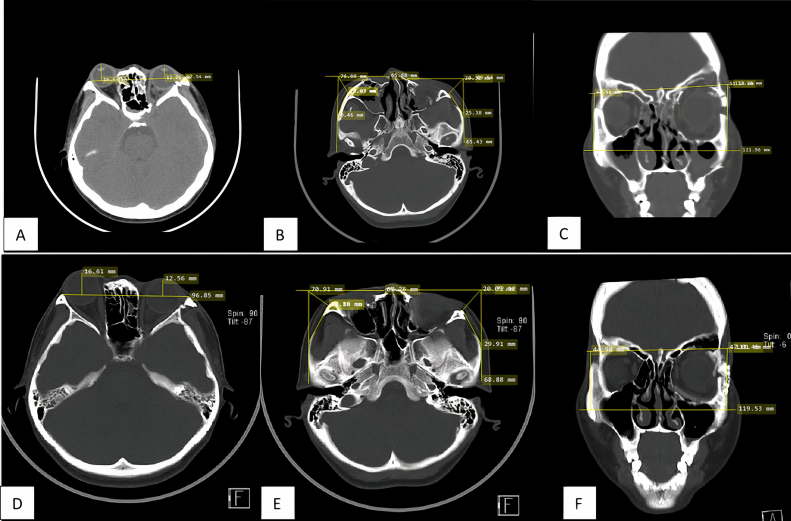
(A) Enophthalmos examination on preoperative CT scan using the Hilal and Trokel method shows enophthalmos in the left eye (with a difference of >2 mm between the two eyes). (B) Malar projection deficit examination. Assessed by axial sections, the width of the zygoma anteriorly and posteriorly was assessed by measuring the distance at that point. There is a deficit between the fracture side and the normal of 1.65 mm. (C) Malar height deficit examination. Coronal sections were assessed by connecting the horizontal line of the upper border of the orbit and the horizontal lines of the right and left zygoma arches. The difference is 7.03 mm compared to the normal side. (D) Enophthalmos examination on postoperative CT scan shows enophthalmos in the left eye (with a difference of >2 mm between the two eyes). (B) Malar projection deficit examination shows a decreasing deficit between the fracture side and the normal side of 1.27 mm. (C) Malar height deficit examination shows a decreasing deficit between the fracture side and the normal side of 2.03 mm.
